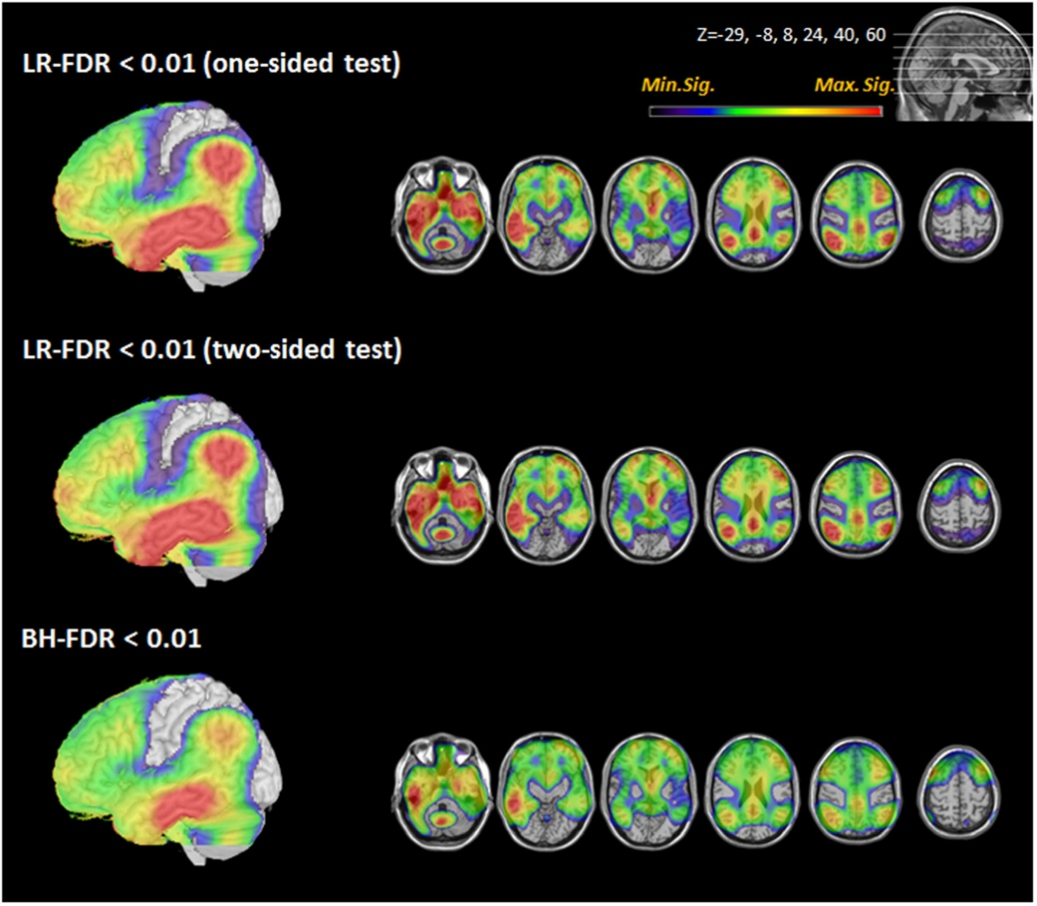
Brain regions with significantly lower FDG uptake in probable AD compared to NC. Regions with lower FDG uptake in probable AD are displayed. The left hemisphere is shown as a 3D volume rendering. The reduction in the FDG uptake in the temporal, parietal, and posterior prefrontal regions was commonly found in the LR-FDR and BH-FDR methods. Extensive hypometabolic areas extending to posterior prefrontal were detected with the LR-FDR one-sided and two-sided tests. The color bar range from minimum to maximum significance level denotes the significance of the likelihood ratio in both LR-FDR methods and of the *p*-value in BH-FDR method. (AD: Alzheimer’s disease; FDR: False discovery rate; LR-FDR: Likelihood ratio false discovery rate; NC: Normal controls).
